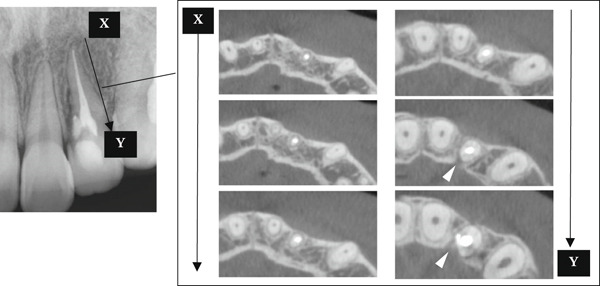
CBCT images of #22 at 24‐month follow‐up. Complete alveolar bone healing is observed except near the palatal cervix (white arrowhead).
